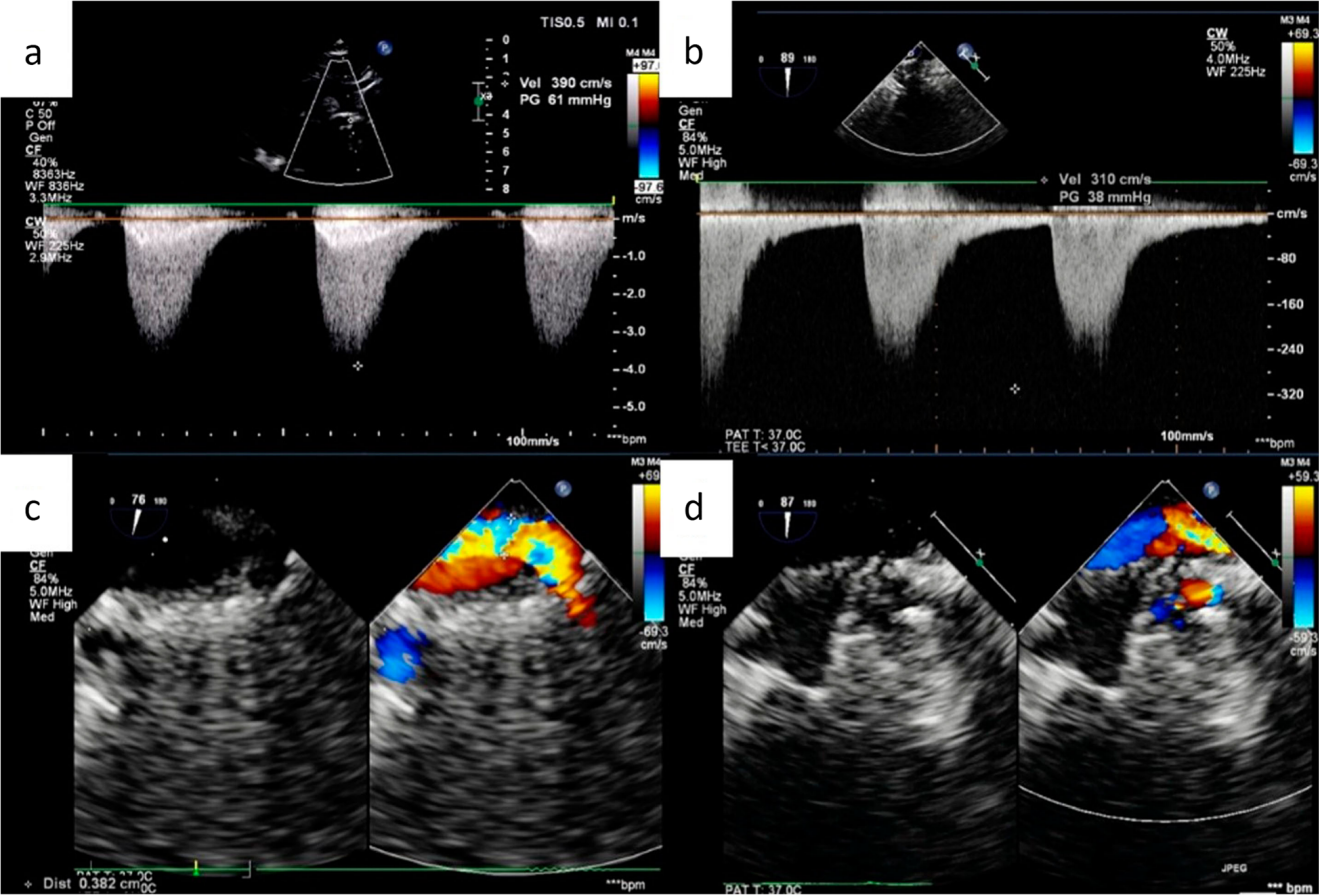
Preoperative transthoracic echocardiography showed the descending aorta with a pressure difference across the thoracic aorta of 61 mmHg (a) and these findings were confirmed by transesophageal echocardiography (b and c). The postoperative echocardiography exhibited abolition of the descending aortic flow rushing with no pressure difference (d)
